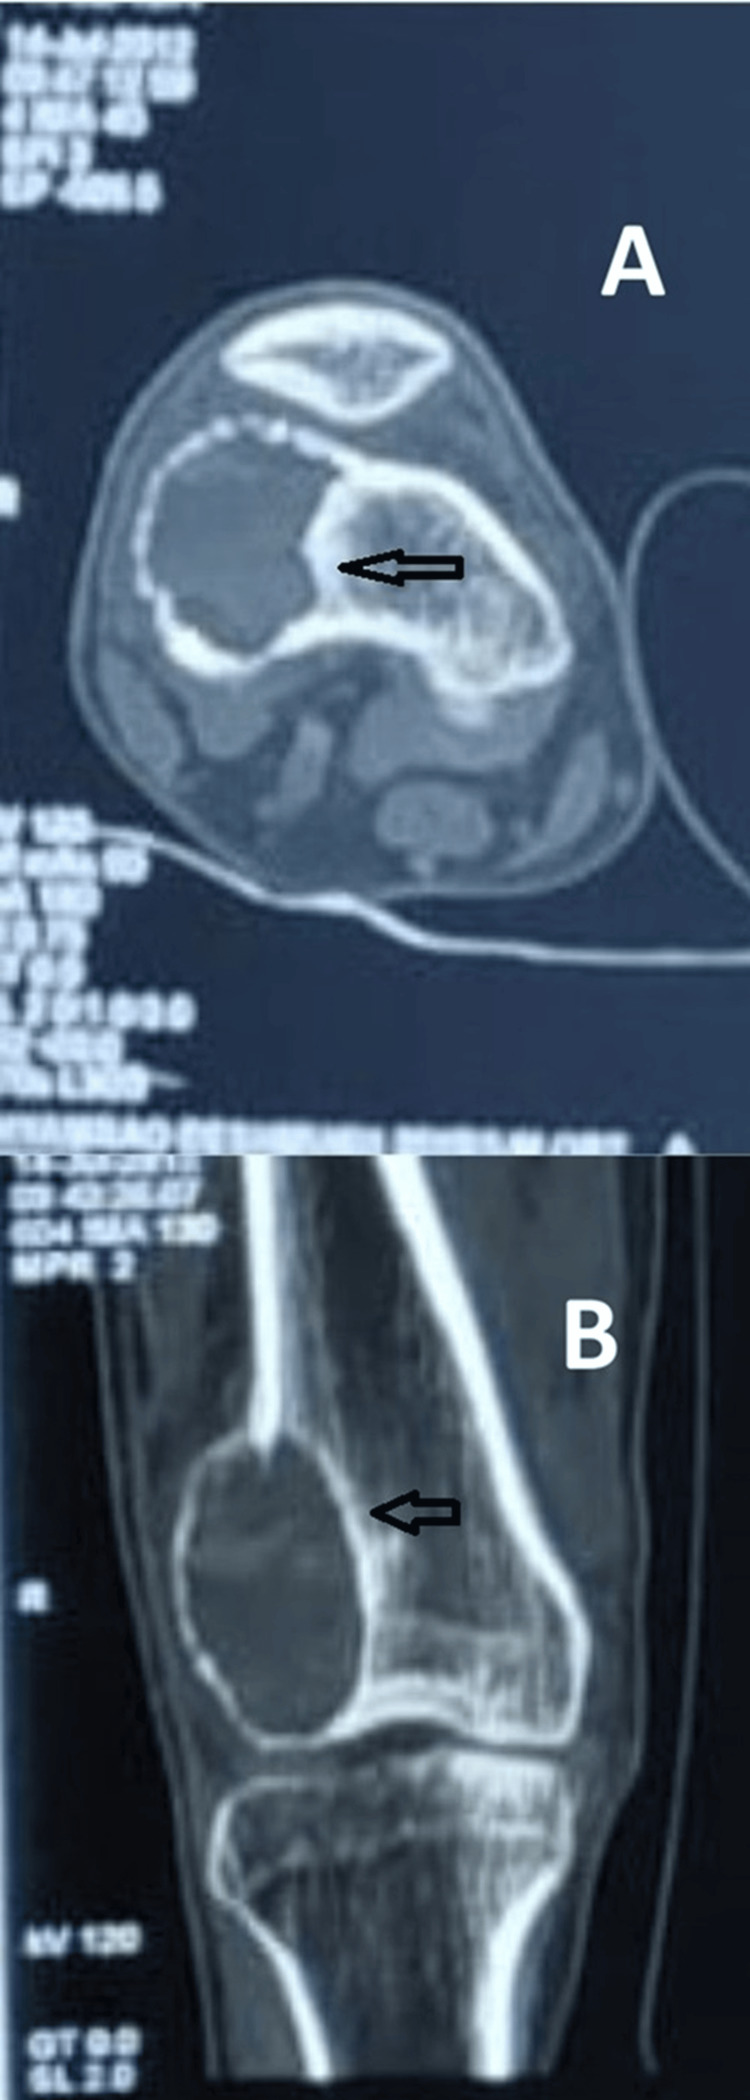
(A) Axial CT scan showing a lytic lesion centered in the right lateral condyle of femur. (B) Coronal CT scan showing cortical erosion in the right distal femur lateral condyle, with the arrow showing the medial margins of the lesion in both the views.
